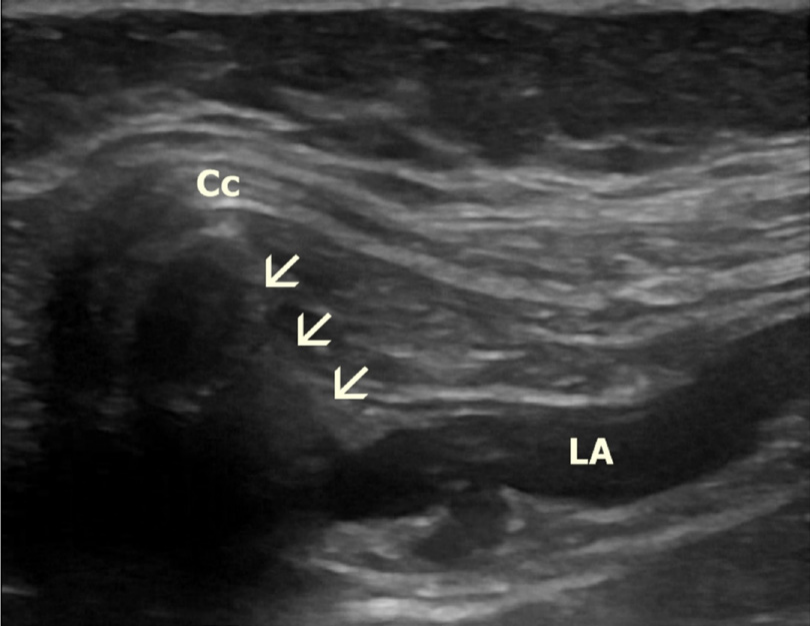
Sonographic visualization of LA. Arrow indicates the needle. Cc, costal cartilage; LA, local anesthetic.
